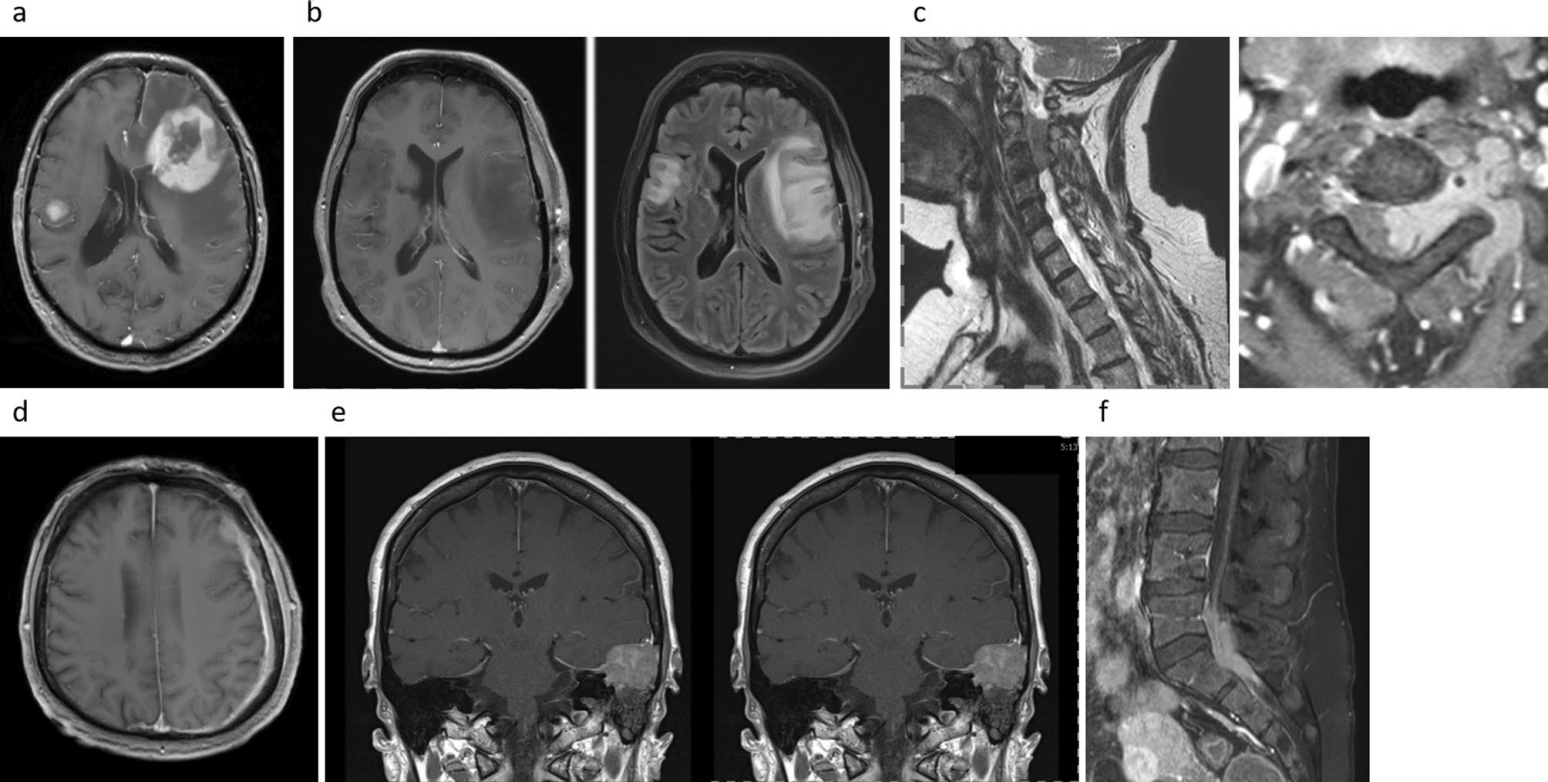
Radiologic aspects of central lymphoma manifestations in our study. a Cerebral DLBCL. T1-weighted (T1-w) 2D FLASH sequence post-contrast. b Intravascular LBCL of small- and medium-sized intracerebral and meningeal vessels. T1-weighted gradient-recalled echo (GRE) post-contrast and T2 FLAIR TSE BLADE sequence. c Spinal dura infiltration of DLBCL. T2-weighted turbo spin-echo (TSE). T1 fat saturated turbo spin-echo (TSE) post-contrast. d Cerebral dura infiltration of DLBCL. T1-weighted (T1-w) 2D FLASH sequence post-contrast. e Bone-associated lymphoma with cerebral infiltration. T1-weighted (T1-w) Turbo Spin-Echo (TSE) sequence post-contrast. f Spinal dura infiltration of DLBCL. Fat-saturated T1-weighted (T1-w) Turbo Spin-Echo (TSE) sequence post-contrast
